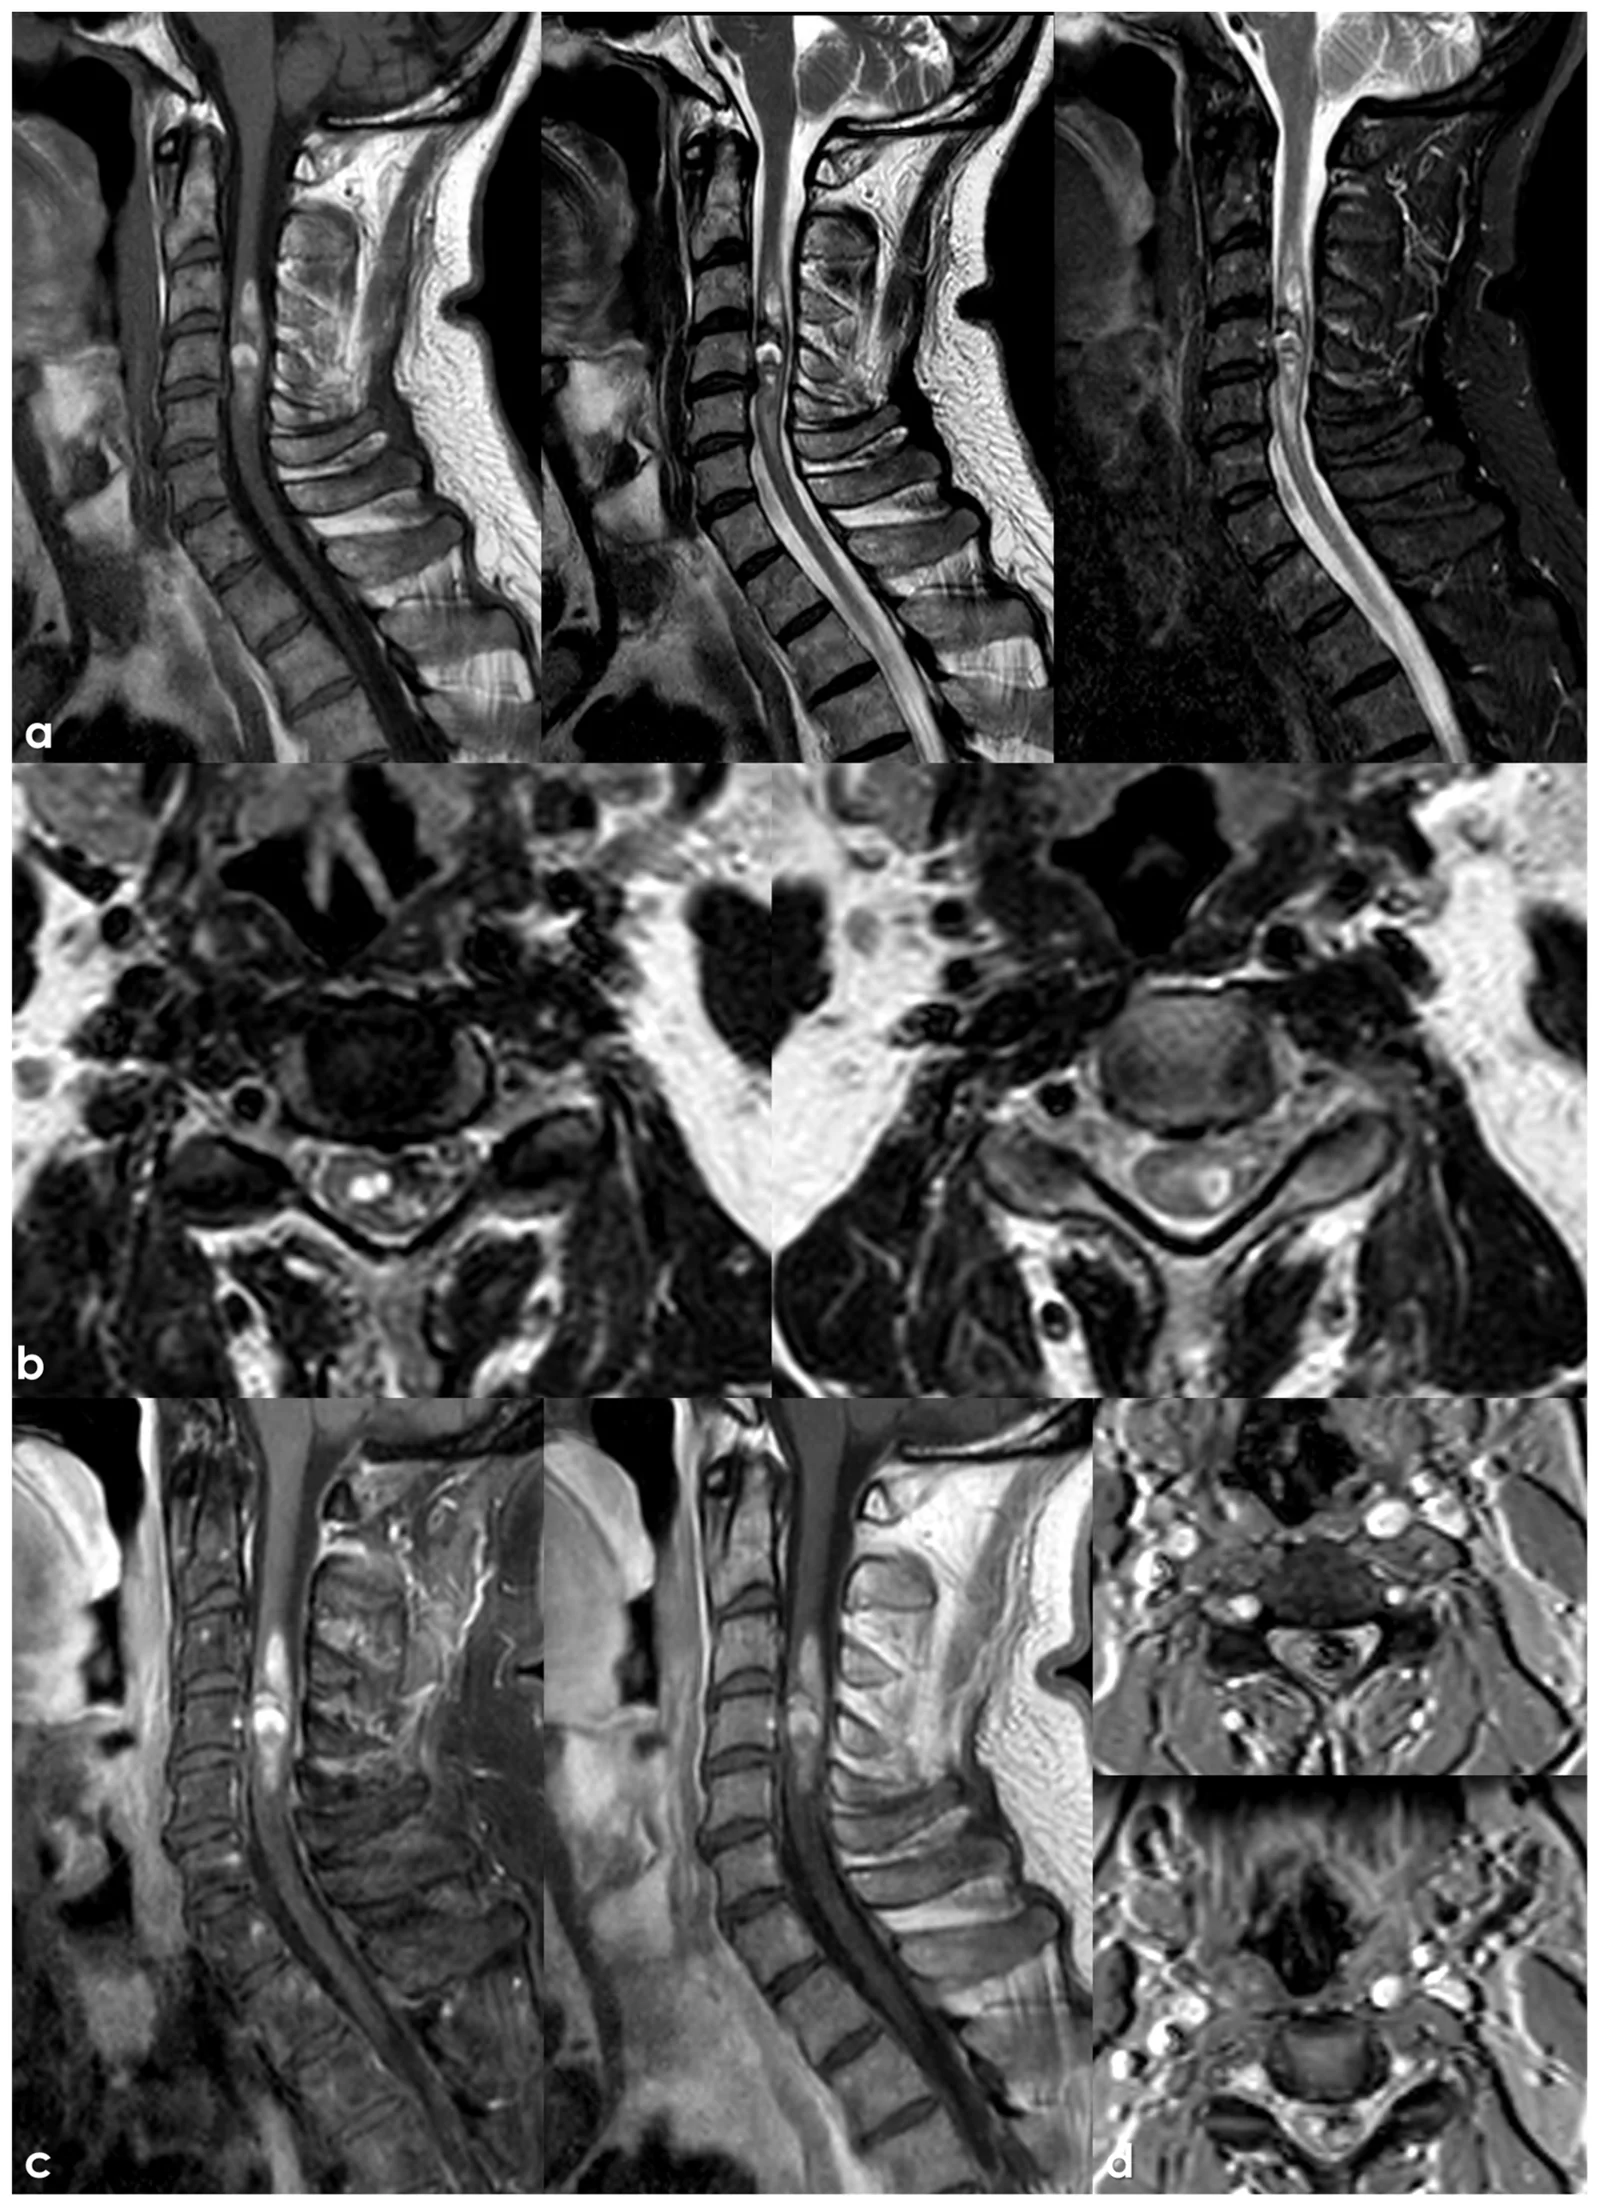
Cervical spine MRI in the same patient of Figure 1. (Panel a): Sagittal T1W, T2W and STIR sequence (from (left) to (right)), confirming a multiloculated rounded hyperintense lesion surrounded by mild edema with tumefactive effect. (Panel b): Axial T2W sequence showing at two contiguous levels the involvement of the lateral funiculus on the right hemicord and of the posterior funiculus on the left hemicord in a relatively deep position. (Panels c,d): Post-contrast T1 Dixon sequence in a sagittal and axial plane. All these findings are consistent for a subacute hemorrhage with an underlying intramedullary cervical cavernous angioma. The sister of the patient received a diagnosis of FCCM a few years before (frameshift mutation of *CCM2* gene) and, at that time, the patient refused to undergo genetic testing, although being highly susceptible to receiving the same diagnosis.
